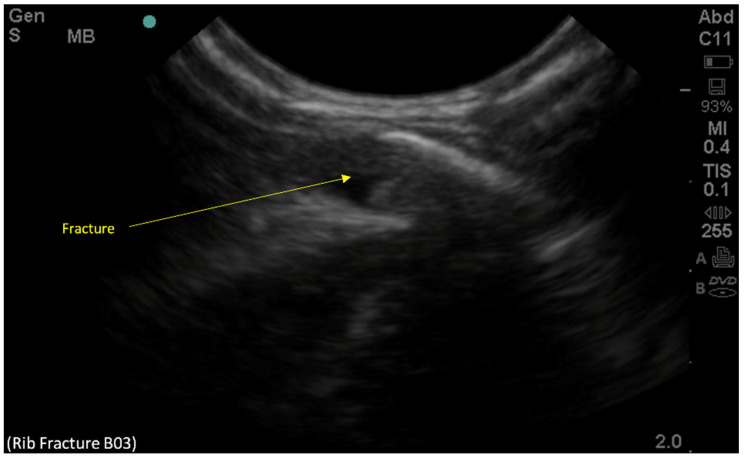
Point-of-care-ultrasound of the left posterior third rib on the longitudinal view demonstrating cortical disruption and suggesting a new fracture of the left posterior third rib, which was not reported in the chest radiographs.
